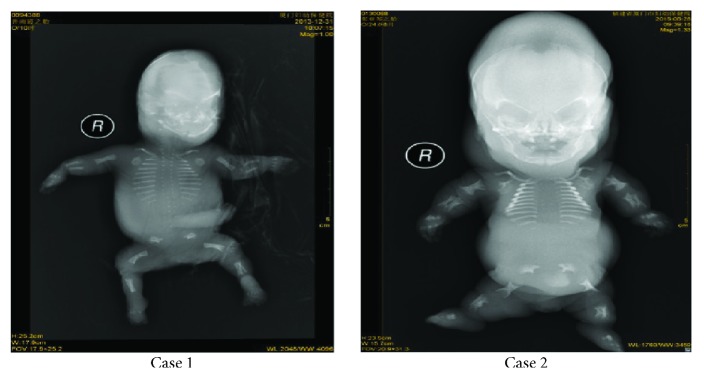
Radiography shows defective skull ossification; vertebral bodies unossified; short, cupped ribs, no fractures; iliac bone ossification limited to the upper part, giving a paraglider-like appearance; long bones, severely short; absence of ossification of all limbs.
